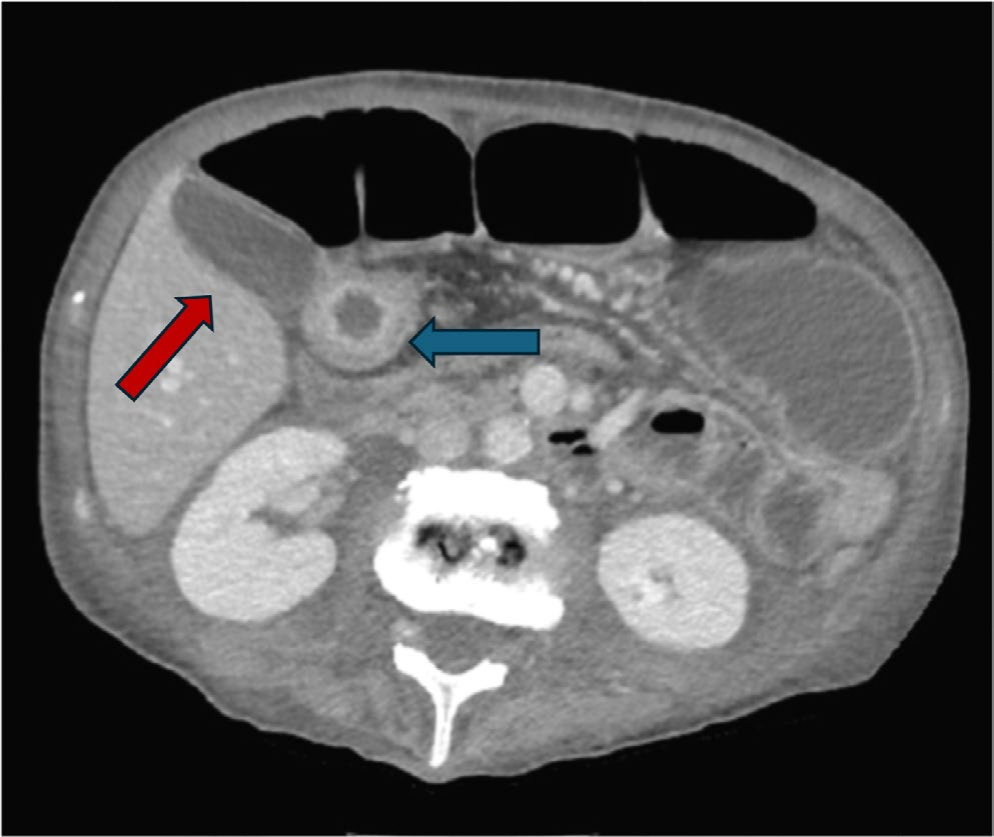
CT scan image demonstrates a thickened loop (blue arrow) in direct contact with the gallbladder wall (red arrow).
